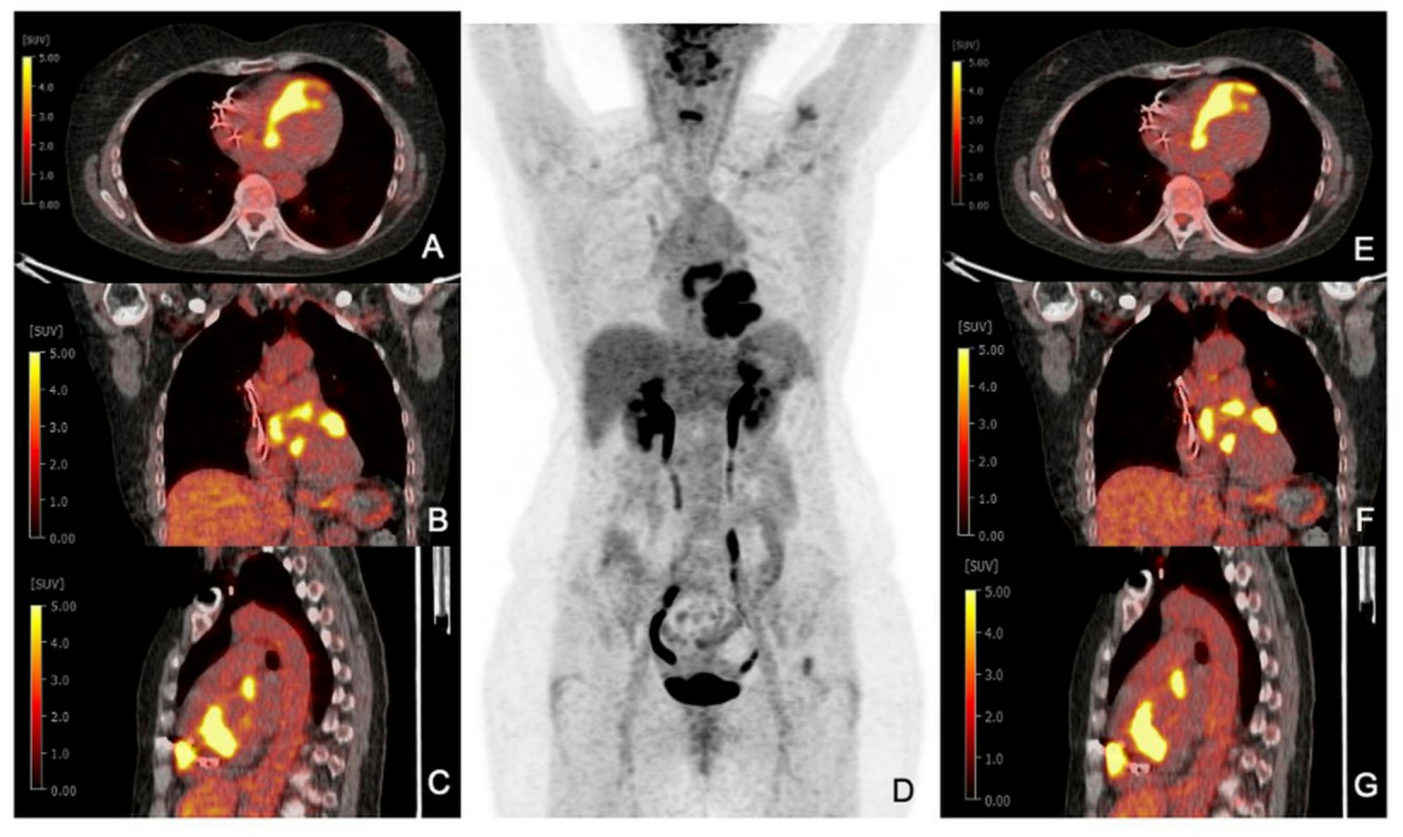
[^18^F]F-FDG PET/CT in patient 28 with suspected isolated CS. A-C transaxial, coronar and sagittal PET/CT fusion after 60 min of FDG uptake time. D maximum intensity projection. E-G transaxial, coronar and sagittal PET/CT fusion after 90 min of FDG uptake time. Intensive focal FDG uptake in the basal and apical septum with involvement of the right ventricle
